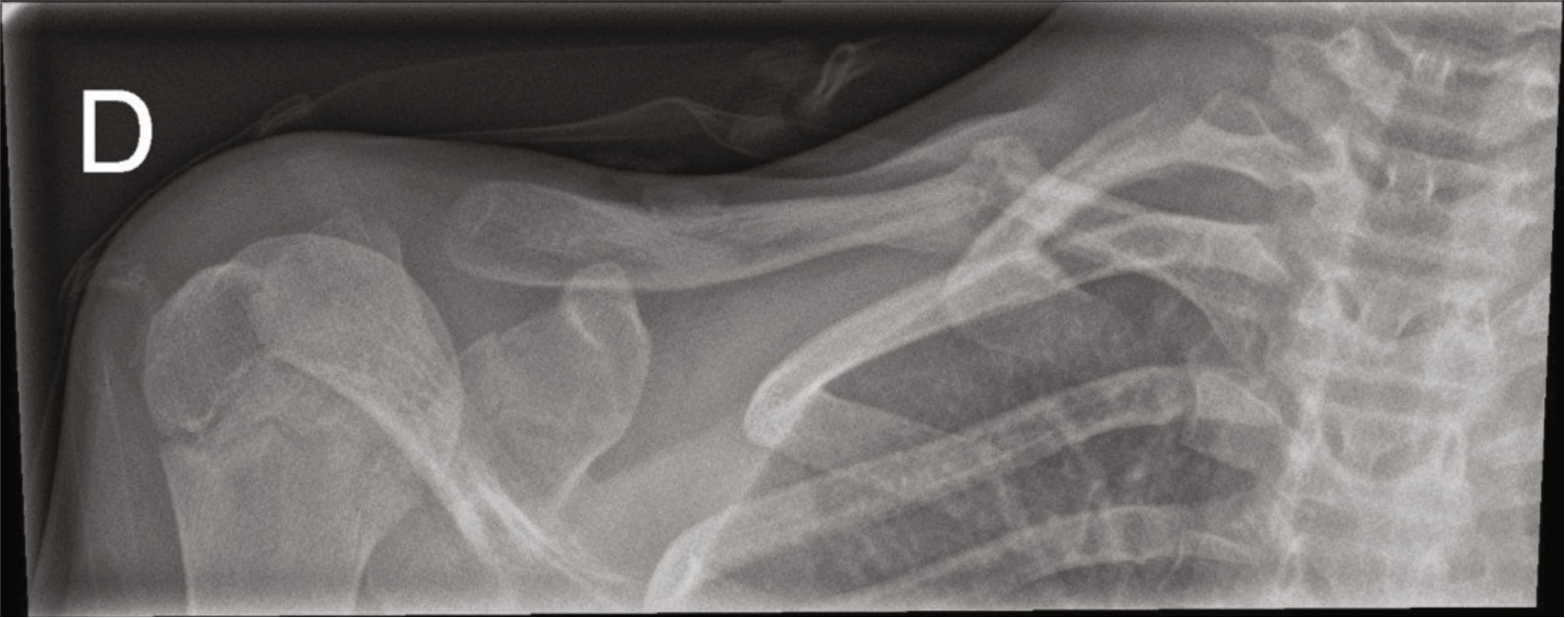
X-ray imaging showing callus formation after three months.
